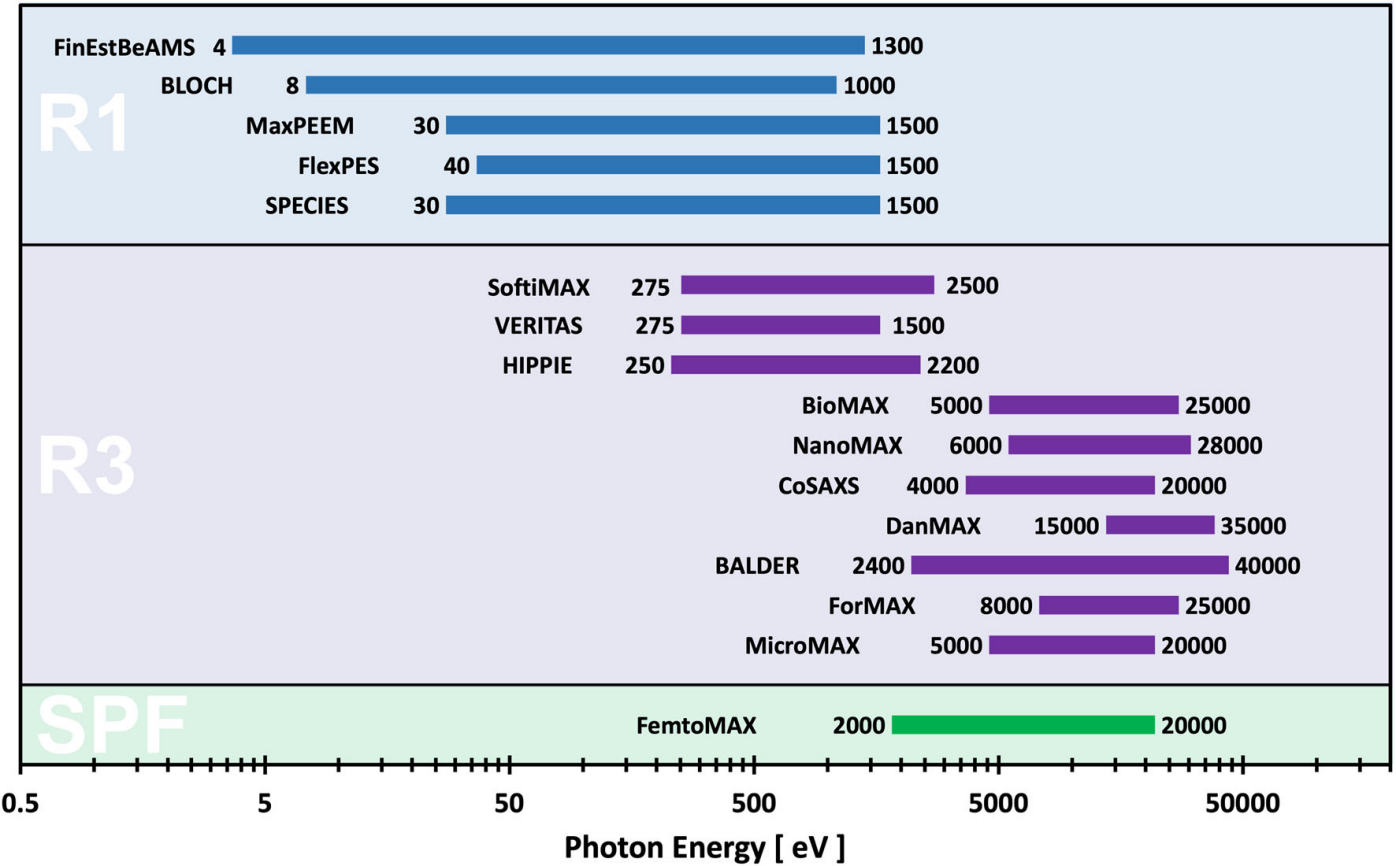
X-ray photon energy range for the 16 MAX IV beamlines on the R1, R3 storage rings, and at the Short Pulse Facility (SPF)
